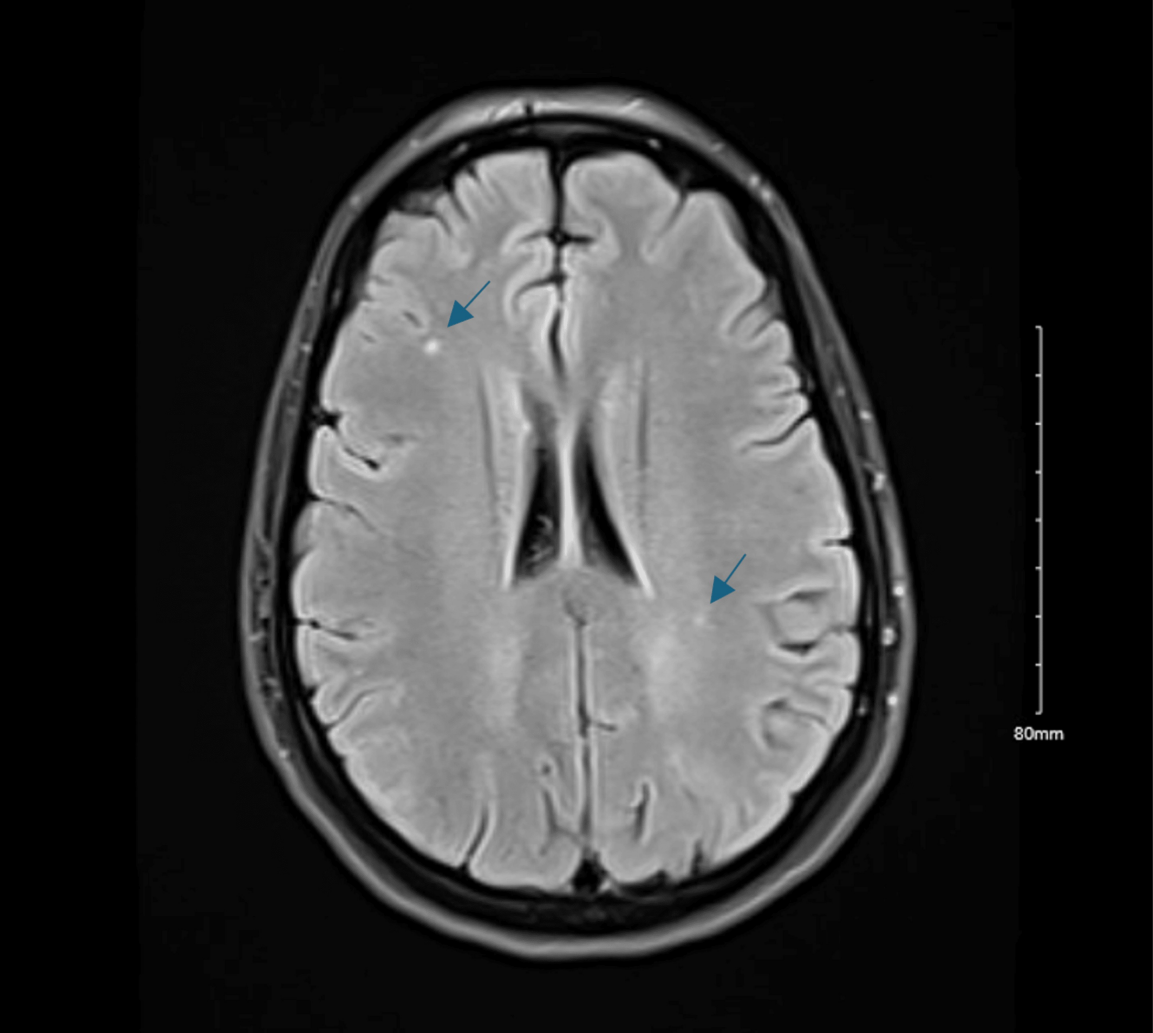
MRI of the Brain Revealing Migraine-Associated White Matter Changes Axial MRI of the brain without contrast demonstrating tiny, nonspecific T2/FLAIR hyperintense foci within the deep white matter of the right frontal and left parietal lobes. These findings are consistent with migraine-related changes. MRI: magnetic resonance imaging; FLAIR: Fluid-attenuated inversion recovery.
